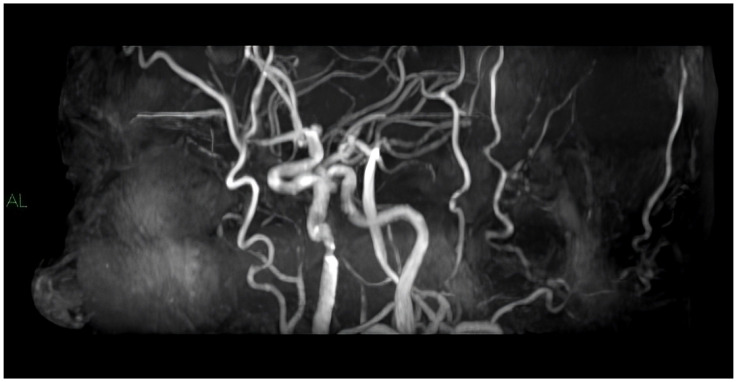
Shown is a 3D MRI reconstruction of a carotid stenosis by a time-of-flight sequence without the use of a contrast medium.
